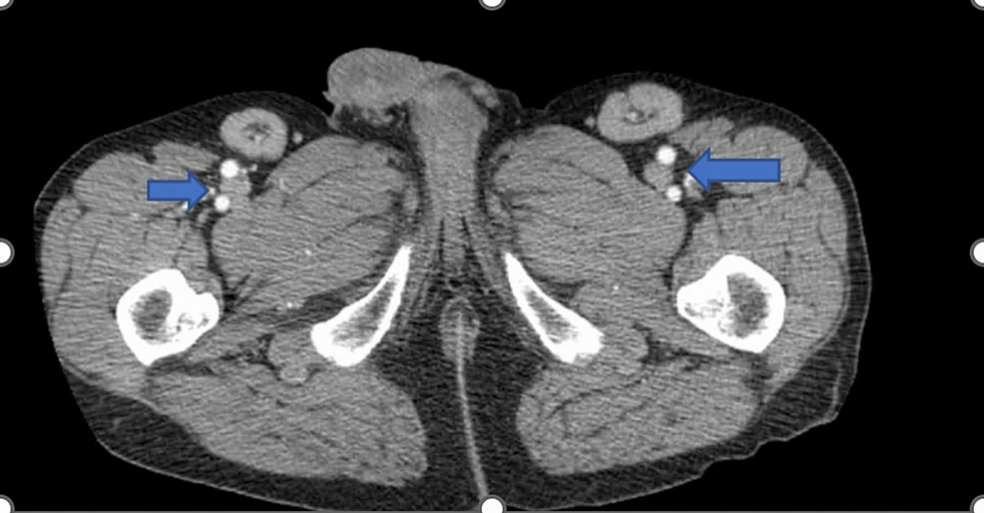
CT chest: bilateral axillary lymphadenopathy (as shown by arrows).
